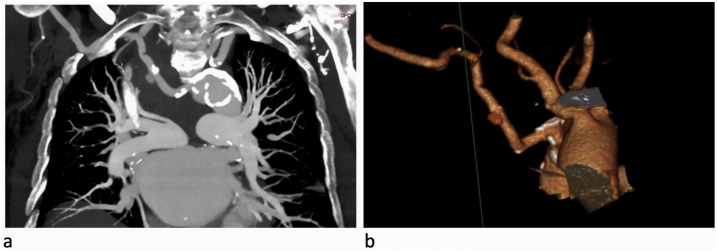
CT scan showing the ARSA and related pseudoaneurysm and the dissection (a); 3D CT reconstruction (b).
